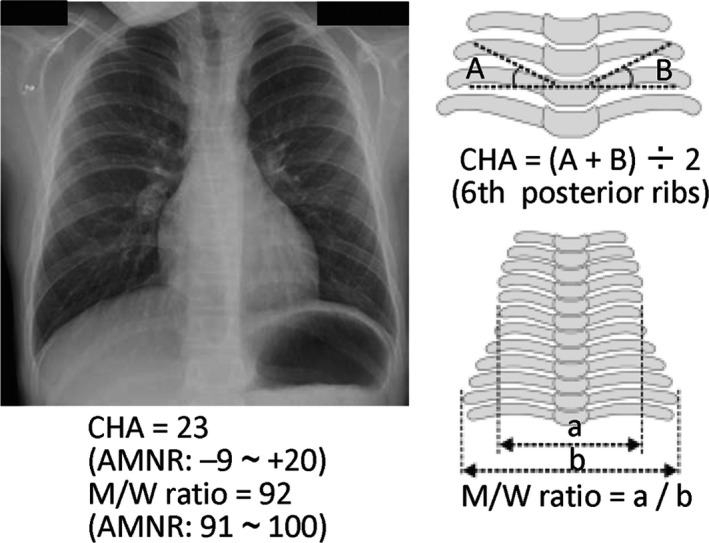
Chest roentgenogram at 9 years of age. AMNR: age‐matched normal range in the Japanese children 3.
